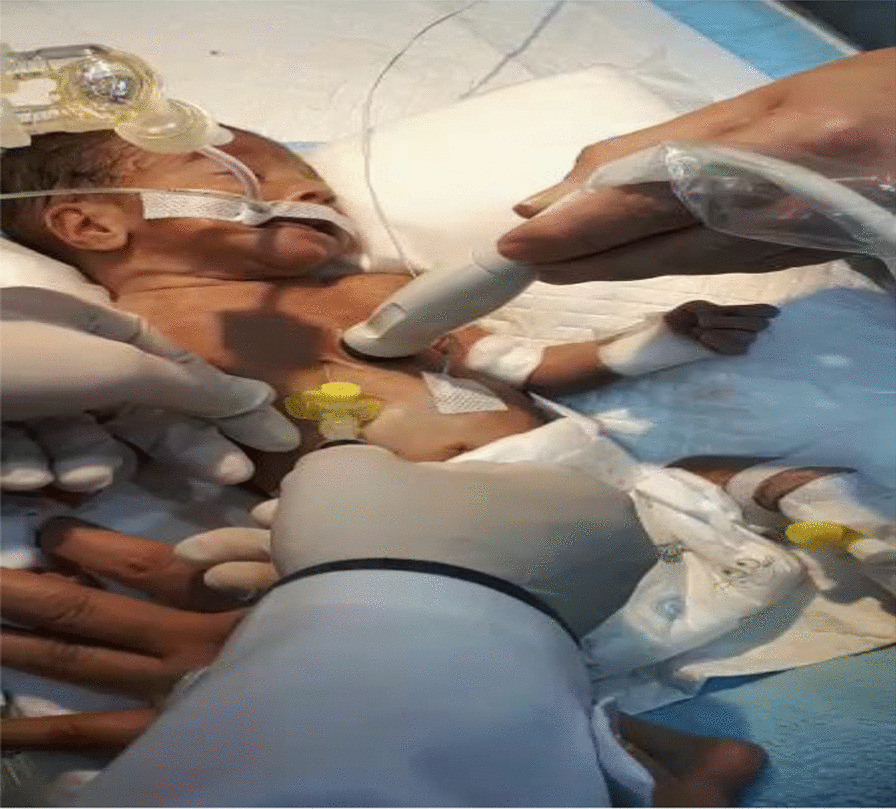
Emergency echocardiography
